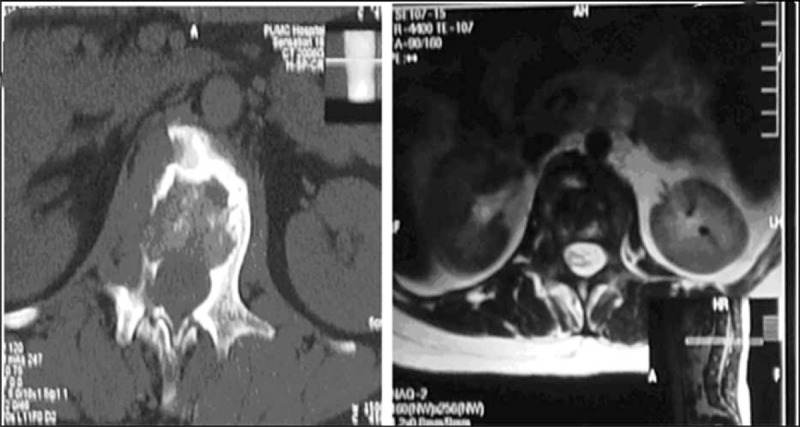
Computed tomography (CT) scan and magnetic resonance imaging (MRI) done in May 2007 collectively pointed toward a left-sided scoliosis with L1 vertebral body destruction resembling tuberculous osteomyelitis.
